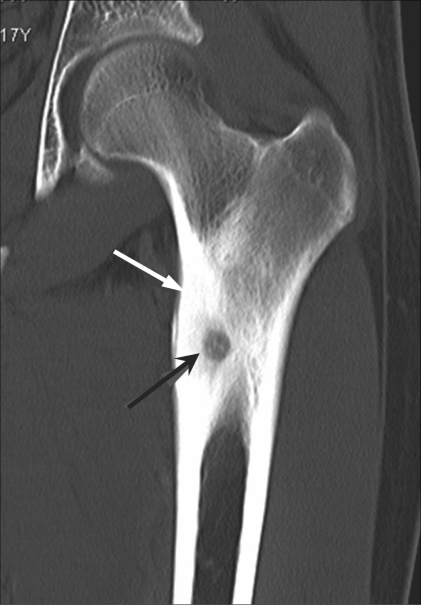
Osteoid osteoma of the femur. Coronal CT scan shows a radiolucent nidus (black arrow) with surrounding bony sclerosis and cortical thickening (white arrow)
